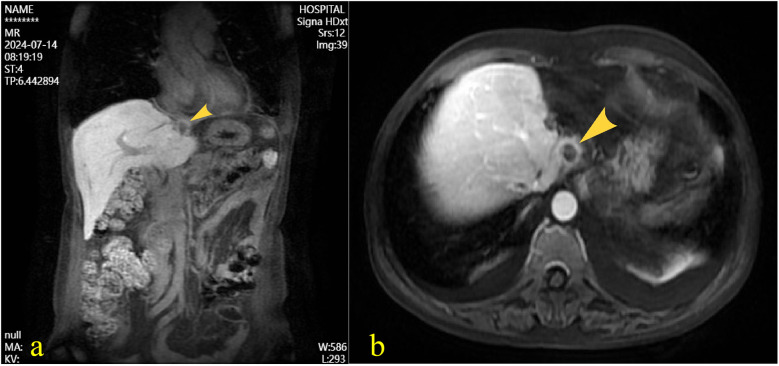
Following intravenous administration of gadoxetate disodium, magnetic resonance imaging of the liver revealed a well-defined roundish abnormal signal near the diaphragmatic surface of the left hepatic lobe. The lesion demonstrated mixed iso-to-hyperintensity on T1-weighted imaging, with signal drop-out on opposed-phase sequences, and iso-to-hyperintense signal on T2-weighted imaging. The longest diameter measured approximately 1.2 cm. (a) coronal view, (b) Axial view.
